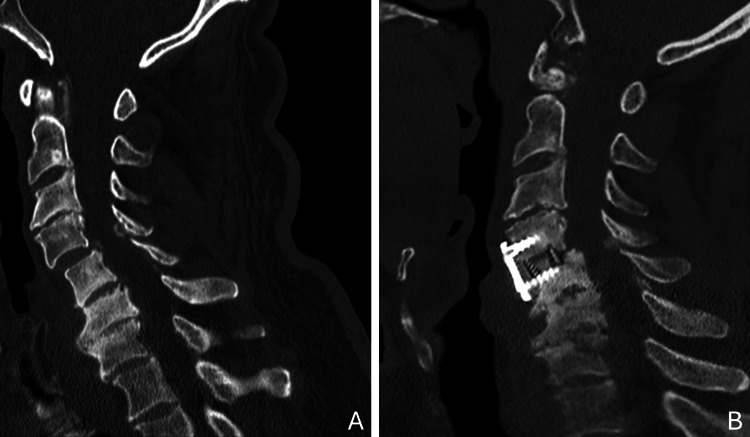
Cervical CT scan Cervical CT scan (sagittal plane) pre-arthrodesis (A) and post-arthrodesis (B)
